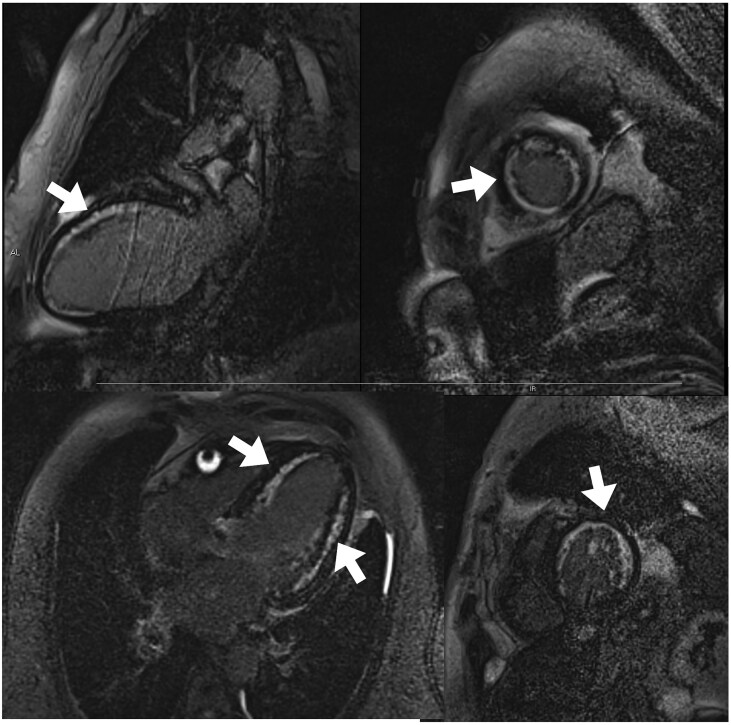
Cardiac MR image. Anterior and apical non-transmural late gadolinium enhancement sequences, 2 and 4-chamber view and basal and mid-short axis (indicated by arrows).
